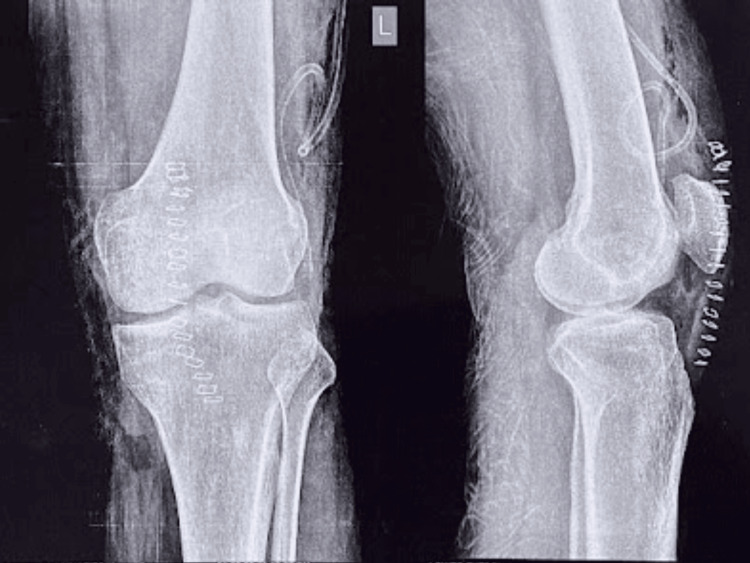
Postoperative plain radiograph of the left knee joint
